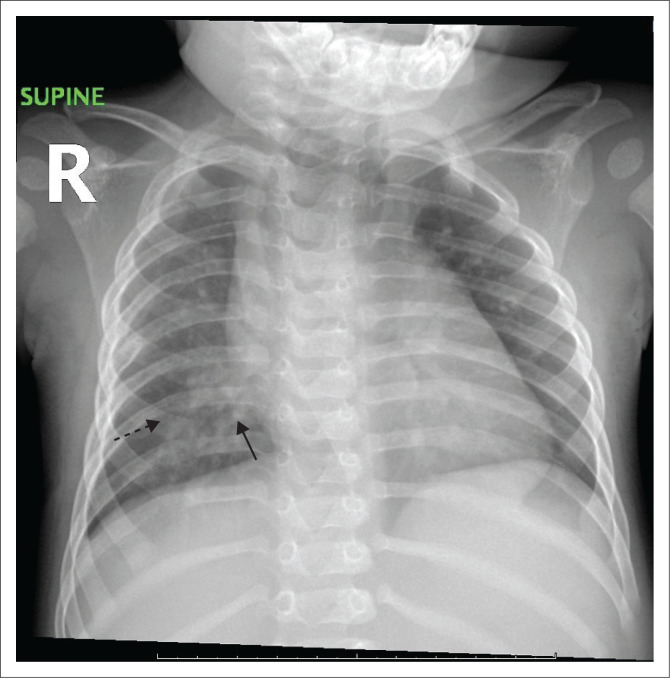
Supine radiograph of an infant showing peribronchial thickening (solid black arrow) and ground-glass opacities (dashed black arrow) involving all lung lobes, indicating diffuse disease.
